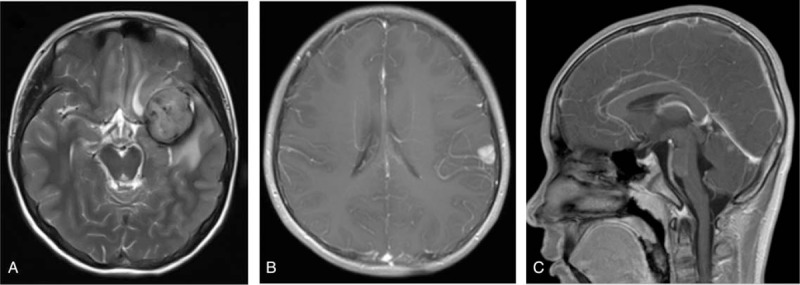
The second MRI scan showing significant enlargement of the lesion in the left temporal lobe (A), and metastatic lesions in the left parietal lobe (B) and the cerebellum (C).
